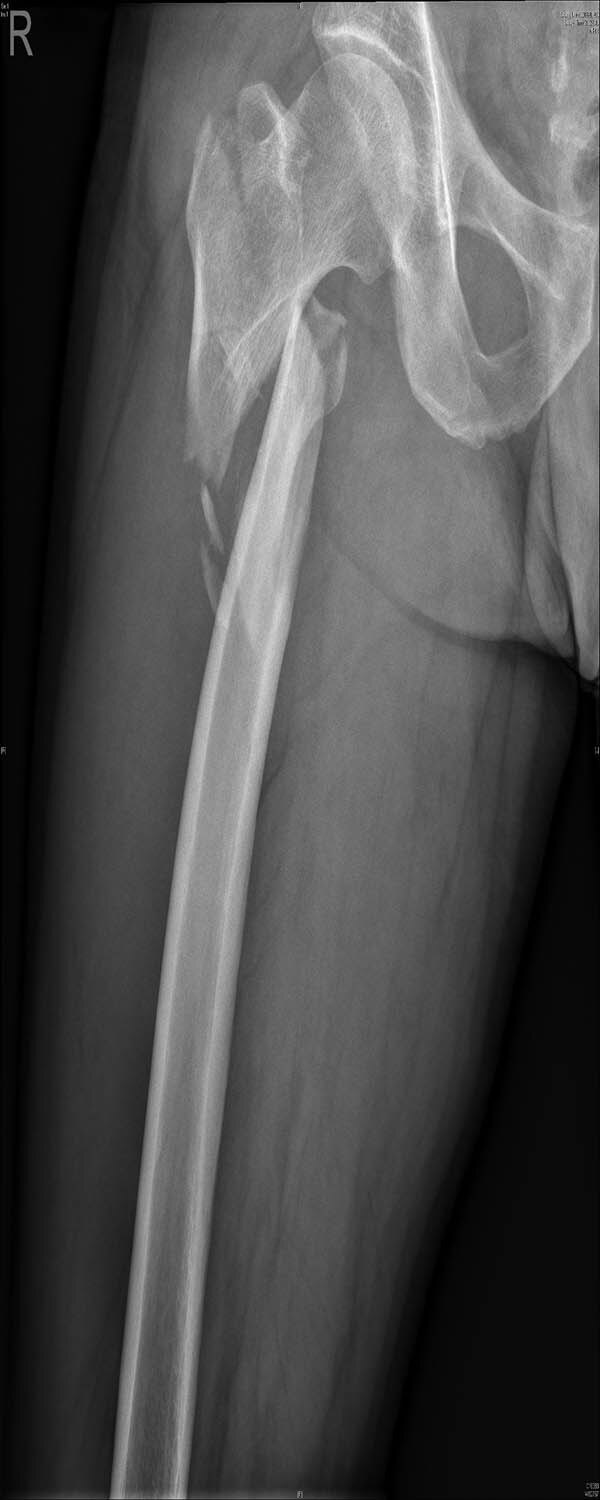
A 42-year-old man fell from height and his X-ray examination revealed that a femoral intertrochanteric fracture had occurred and that the fracture was displaced.
